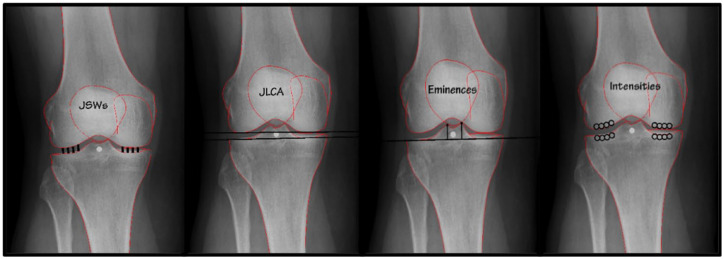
Measured parameters on knee radiographs, from left to right: Joint space width (JSW), joint line convergence angle (JLCA), eminence heights, and subchondral bone intensities.
